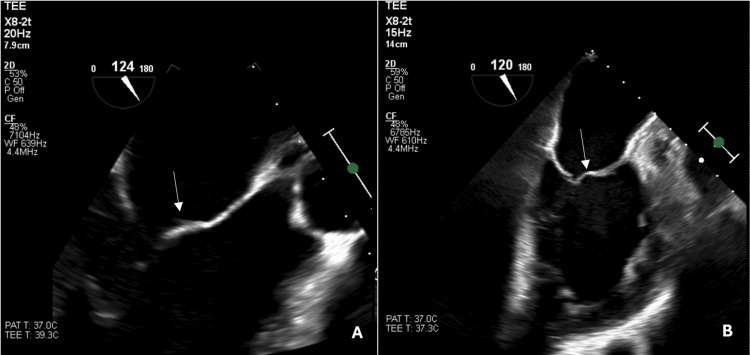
Transesophageal echocardiogram showing (A) a mobile linear vegetation on the anterior leaflet of the mitral valve and (B) the resolution of the mitral valve vegetation post-treatment
